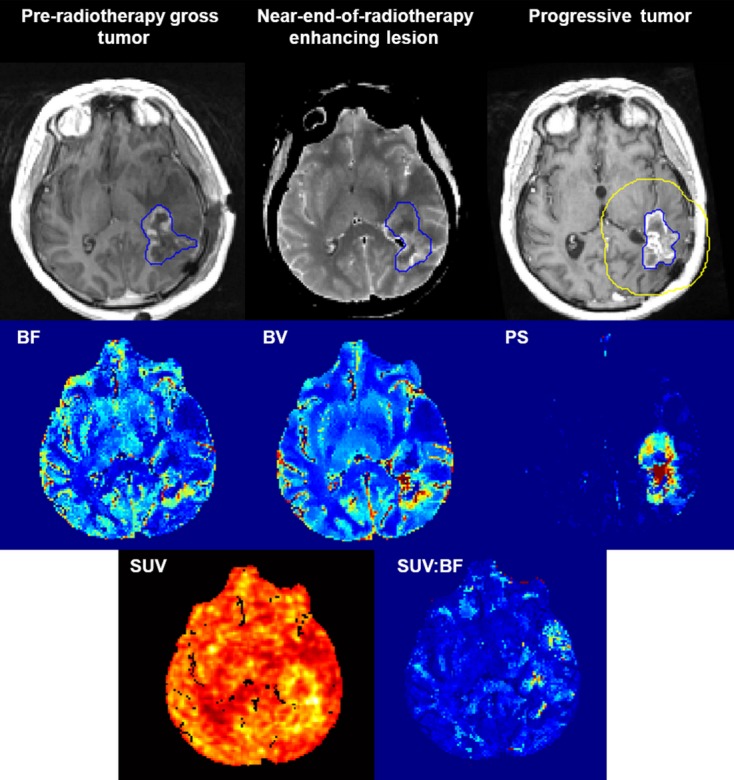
Pre-radiotherapy gross tumour (T1-weighted MR), near-end-of-radiotherapy enhancing lesion (averaged CT) and progressive tumour (T1-weighted MR); and the corresponding parametric maps of blood flow (BF), blood volume (BV), permeability-surface area (PS) product, standard uptake value (SUV) and SUV:BF acquired using CT perfusion and FDG-PET. Blue outlines show the contrast-enhancing lesions delineated by a radiation oncologist. Yellow outline is the 2-cm bounding box that was set for performing logistic regression. MR, magnetic resonance; CT, computed tomography; FDG-PET, 18-Fluorodeoxyglucose positron emission tomography.
